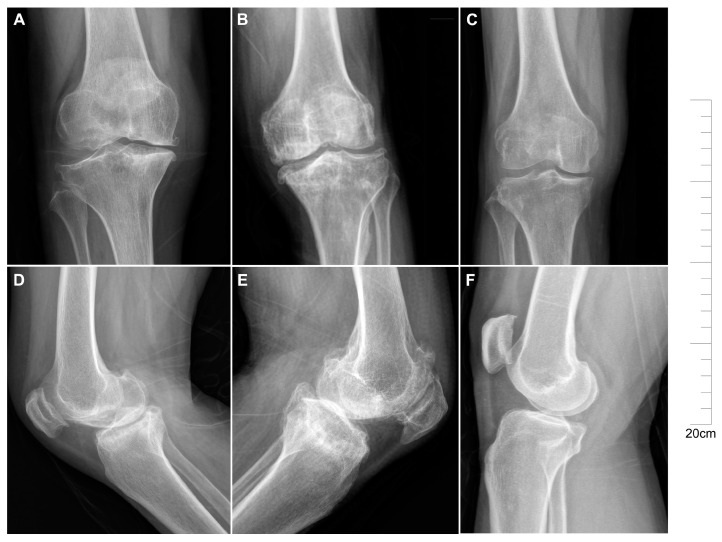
Characteristics of knee joint damage in samples of Kashin–Beck disease and osteoarthritis. Frontal X-ray of the knee joint in KBD (A), OA (B), and normal control (C) subjects. Lateral X-ray of the knee joint in KBD (D), OA (E), and normal control (F) subjects.
